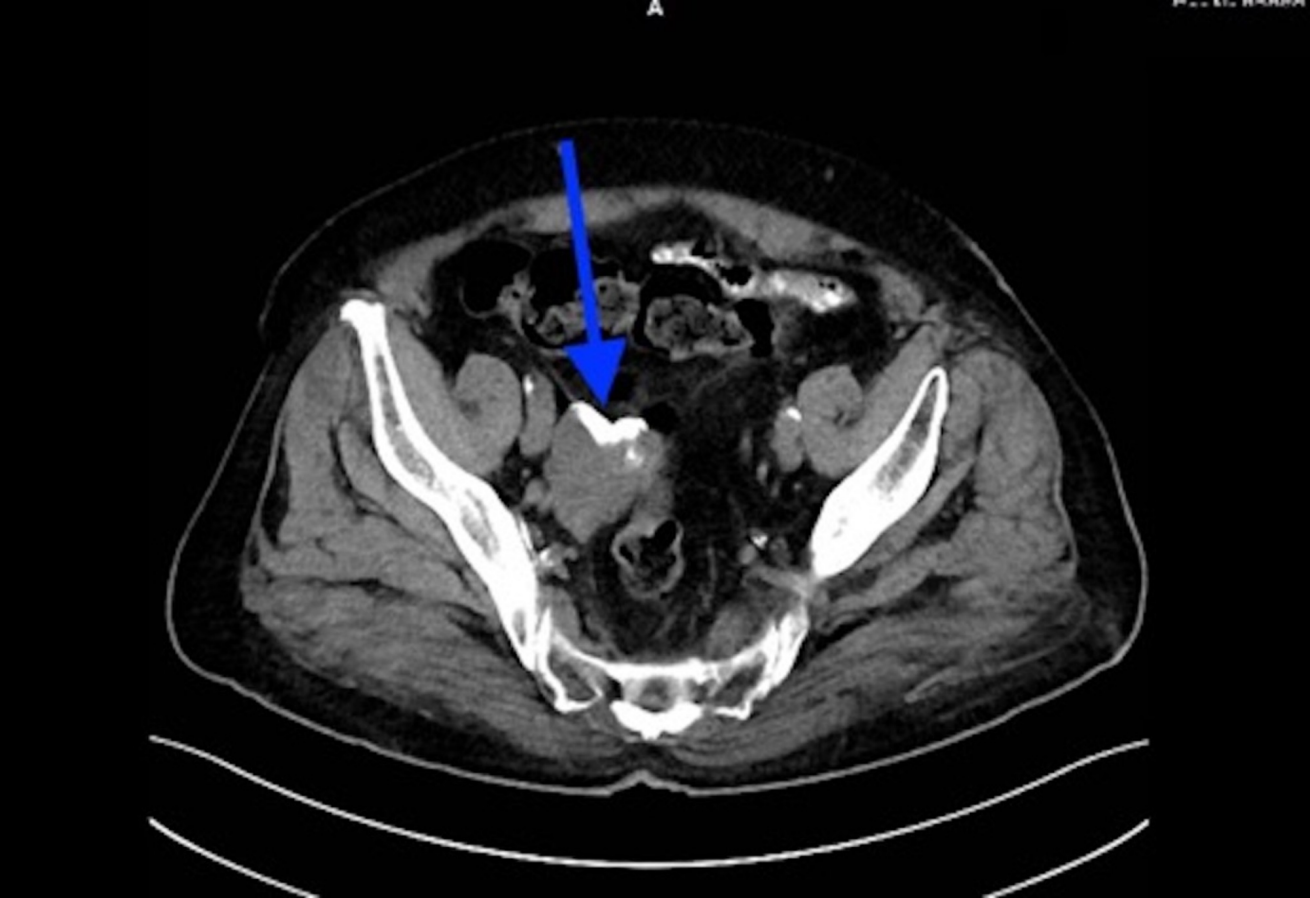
Computed tomography image, 5 x 4 cm calcified mass (blue arrow)
